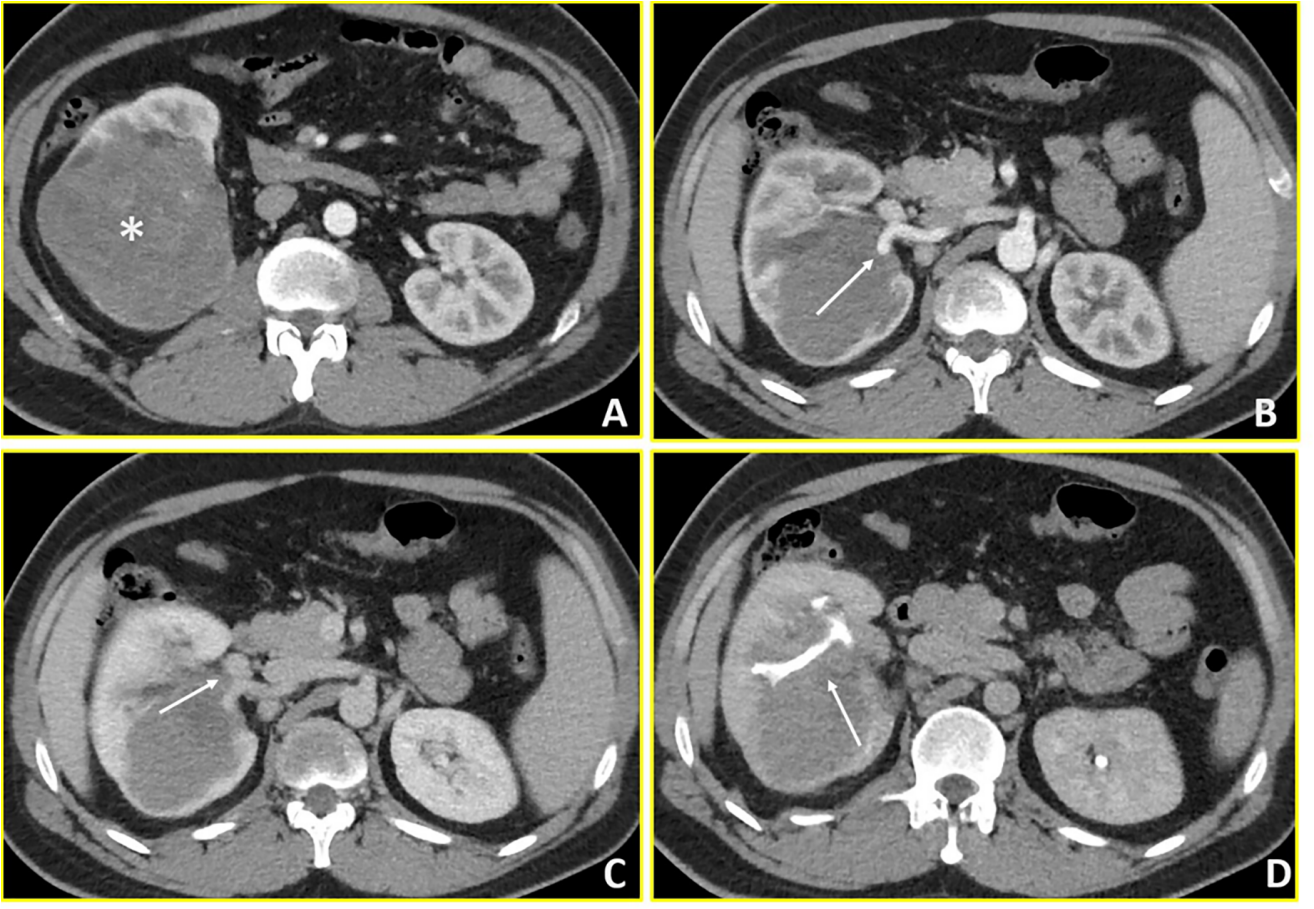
Wilms’ Tumour (Case 1). Contrast-enhanced CT imaging demonstrates a hypovascular mass in the right kidney with extensive vascular and collecting system involvement. (A, B) Axial arterial-phase images reveal a predominantly hypovascular renal mass (asterisk) with infiltration of the right renal artery (arrow in B). (C) Axial venous-phase image shows tumor extension into the right renal vein (arrow). (D) Axial urographic-phase image demonstrates infiltration of the renal collecting system with involvement of the renal calyces (arrow).
